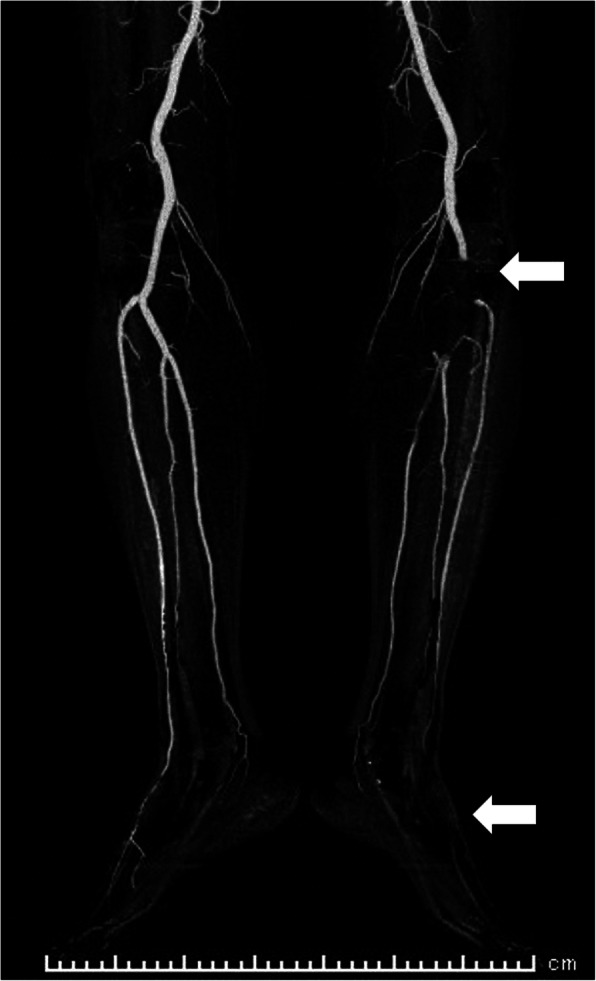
Computed tomography angiography image of the lower extremities taken at acute limb ischemia onset. The upper arrow indicates that the left popliteal artery is occluded, and the lower arrow indicates that the left dorsalis pedis artery cannot be seen clearly
